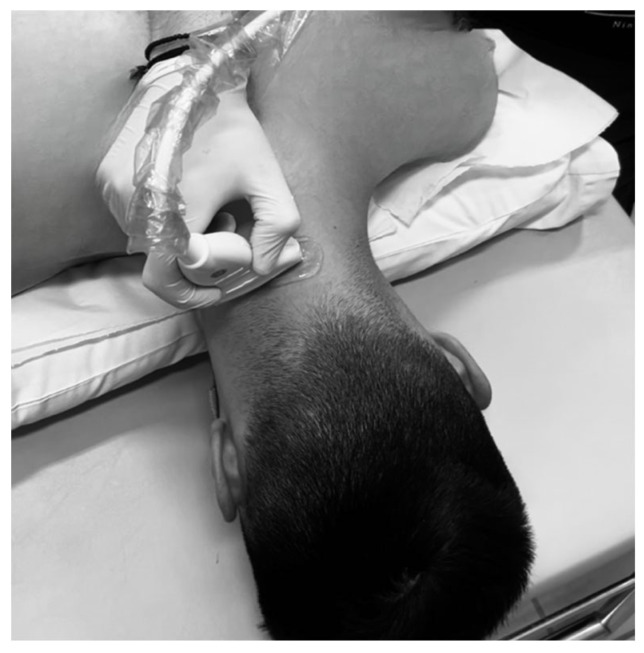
Ultrasonographic measurements of the posterior neck muscles.
